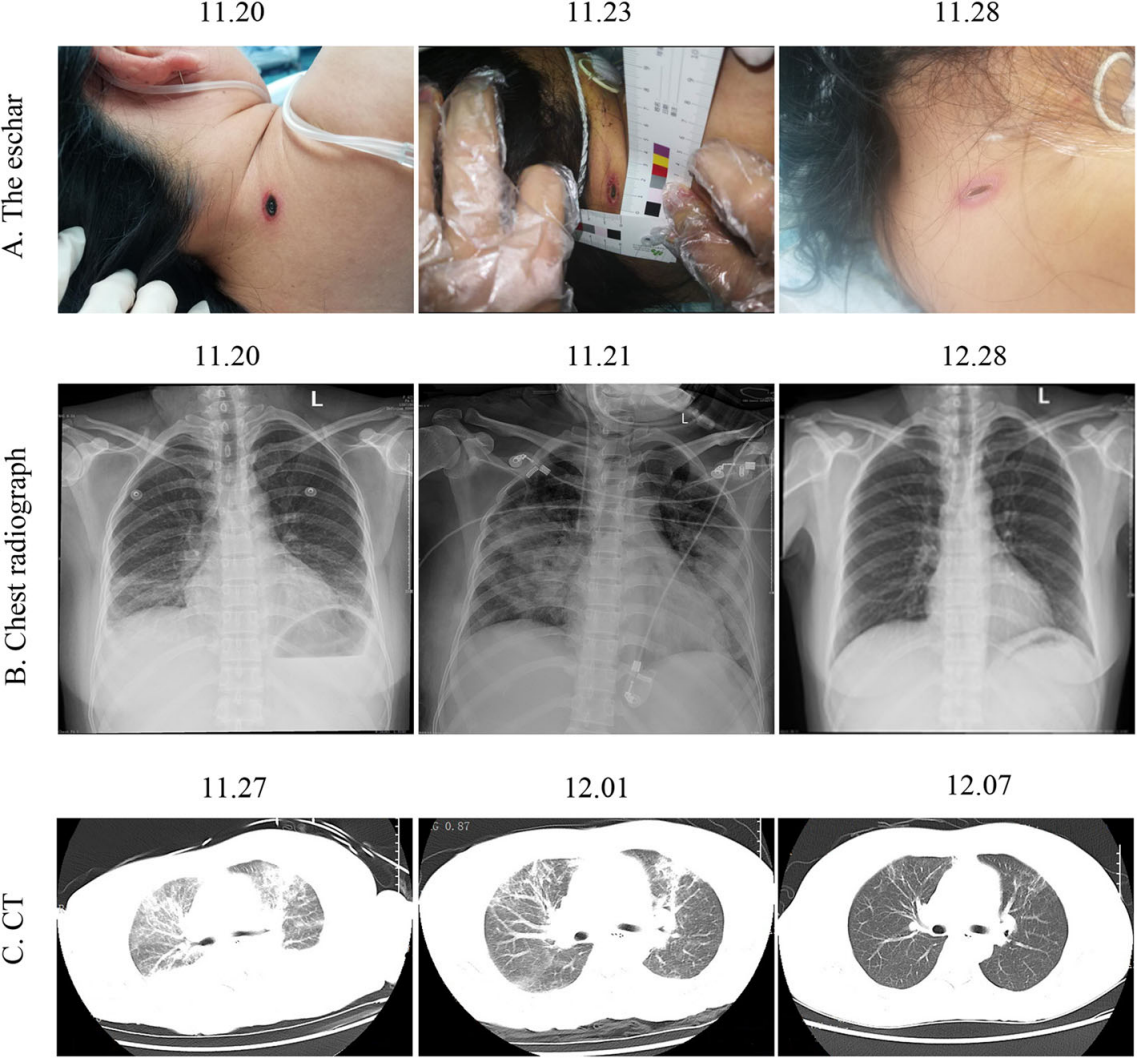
Changes in diagnostic examinations during treatment. a The change in the eschar during treatment. b The change in chest radiograph during treatment. c The change in computed tomography during treatment
